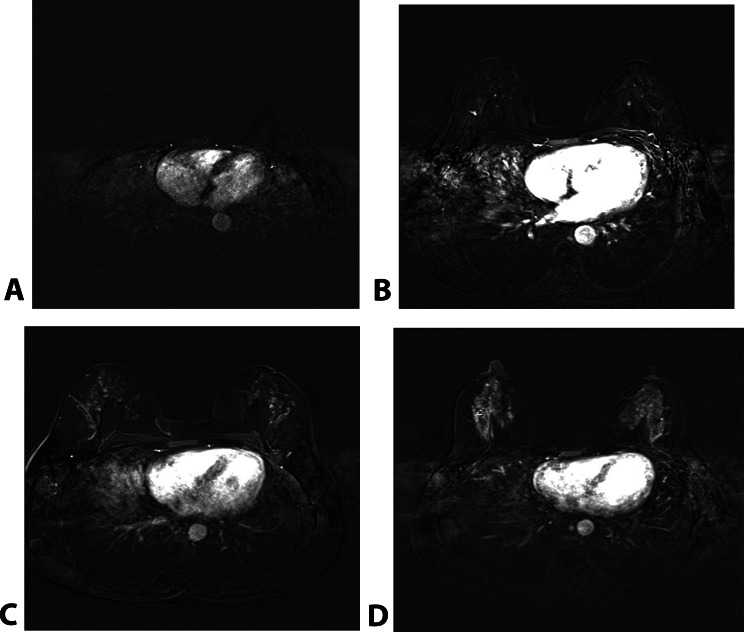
T1-weighted fat-suppressed first contrast-enhanced subtraction MRI scans depicting different levels of BPE (A) Minimal BPE, (B) mild BPE, (C) moderate BPE, and (D) marked BPE
